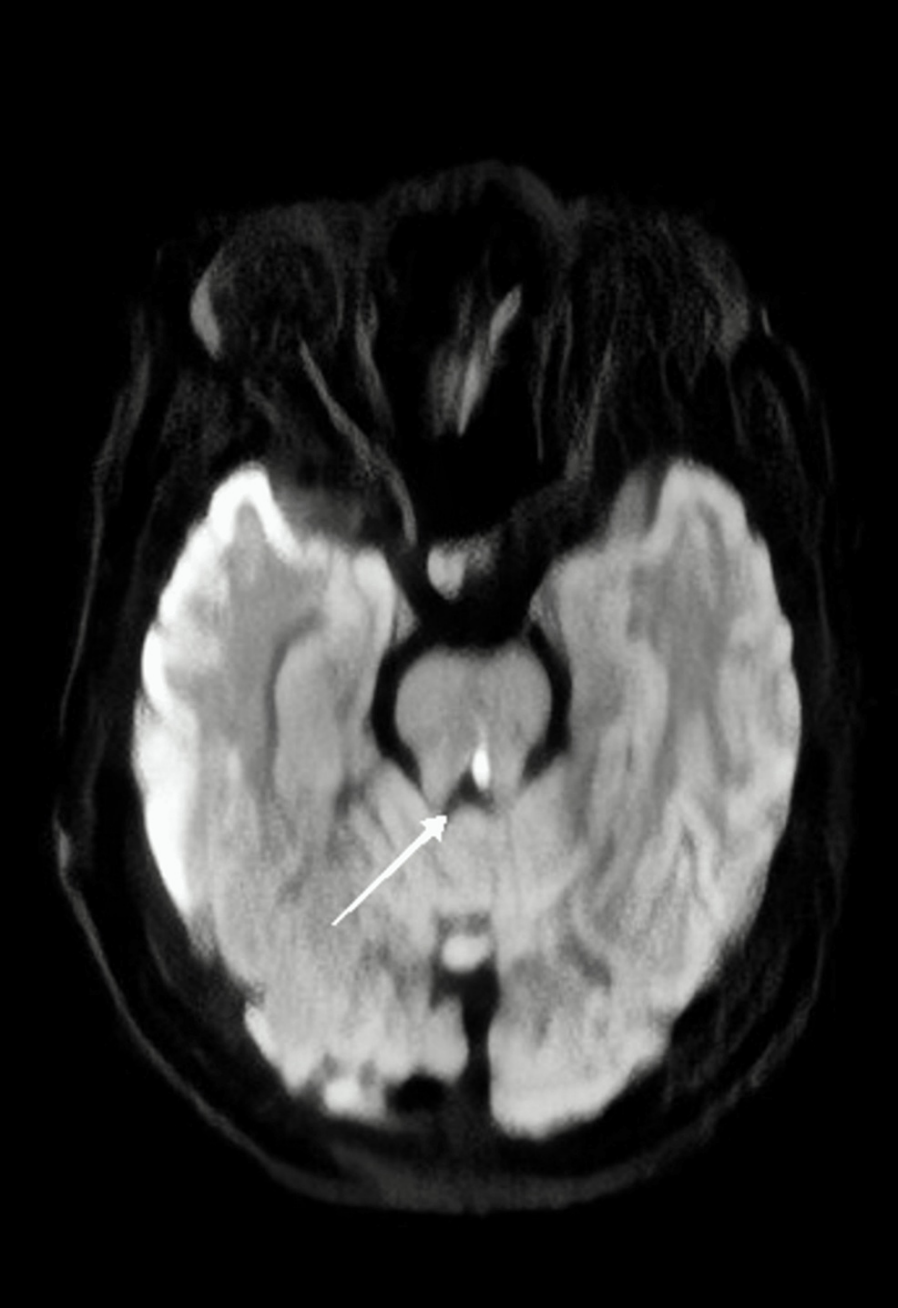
Magnetic resonance imaging of the brain reveals a focus of hyperintensity in the midbrain suggestive of acute infarction (white arrow).
